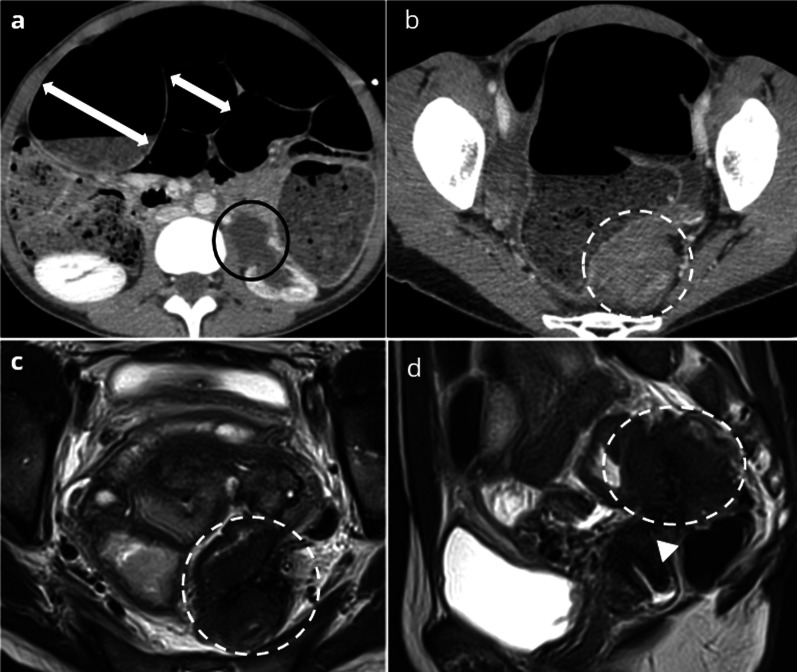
39-year-old female presenting with acute clinical intestinal obstruction. Axial enhanced CT (a, b) shows a large and small bowel distension (arrows), as a consequence of obstruction by a recto-sigmoid mass (white dotted circle, (b)). Features of long-standing left urinary obstruction (black plain circle) may be suggestive of deep endometriosis (a). T2W MRI (c, d) shows a stellar retractile hypoT2 mass of the Douglas pouch with colic asymmetric muscular thickening (white dotted circle) consistent with deep endometriosis of the torus (arrowhead) extending transmurally to the recto-sigmoid with stenosis and left ureter involvement
